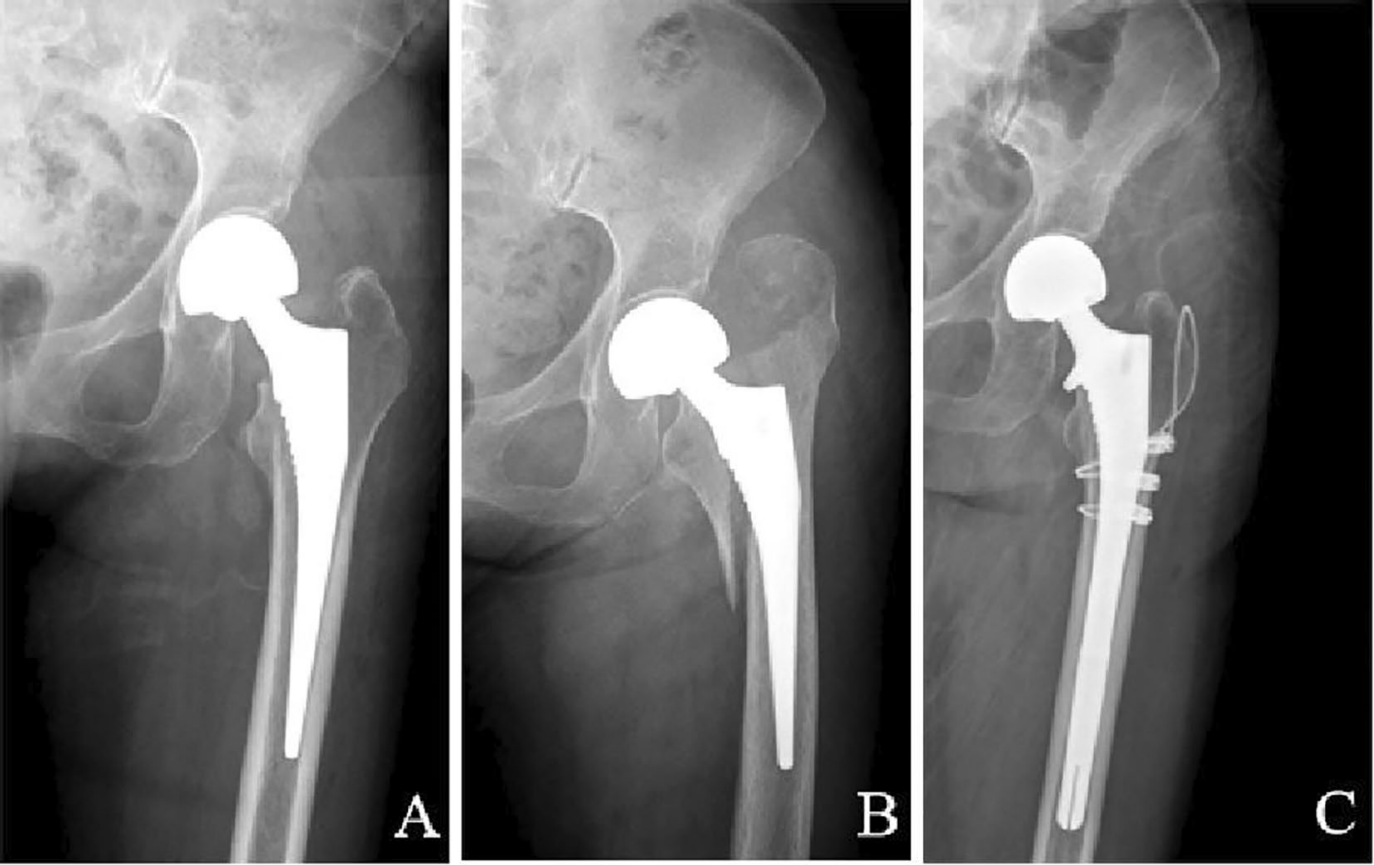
Case 8, an 81-year-old woman. (A) Anterior–posterior radiograph after hemi-arthroplasty. (B) After a fall in the ward, the continuity of the left medial femoral cortex was disrupted on anterior–posterior and lateral radiographs. (C) Postoperative anterior–posterior radiograph shows the patient with a longer stem revision and internal fixation with cables.
